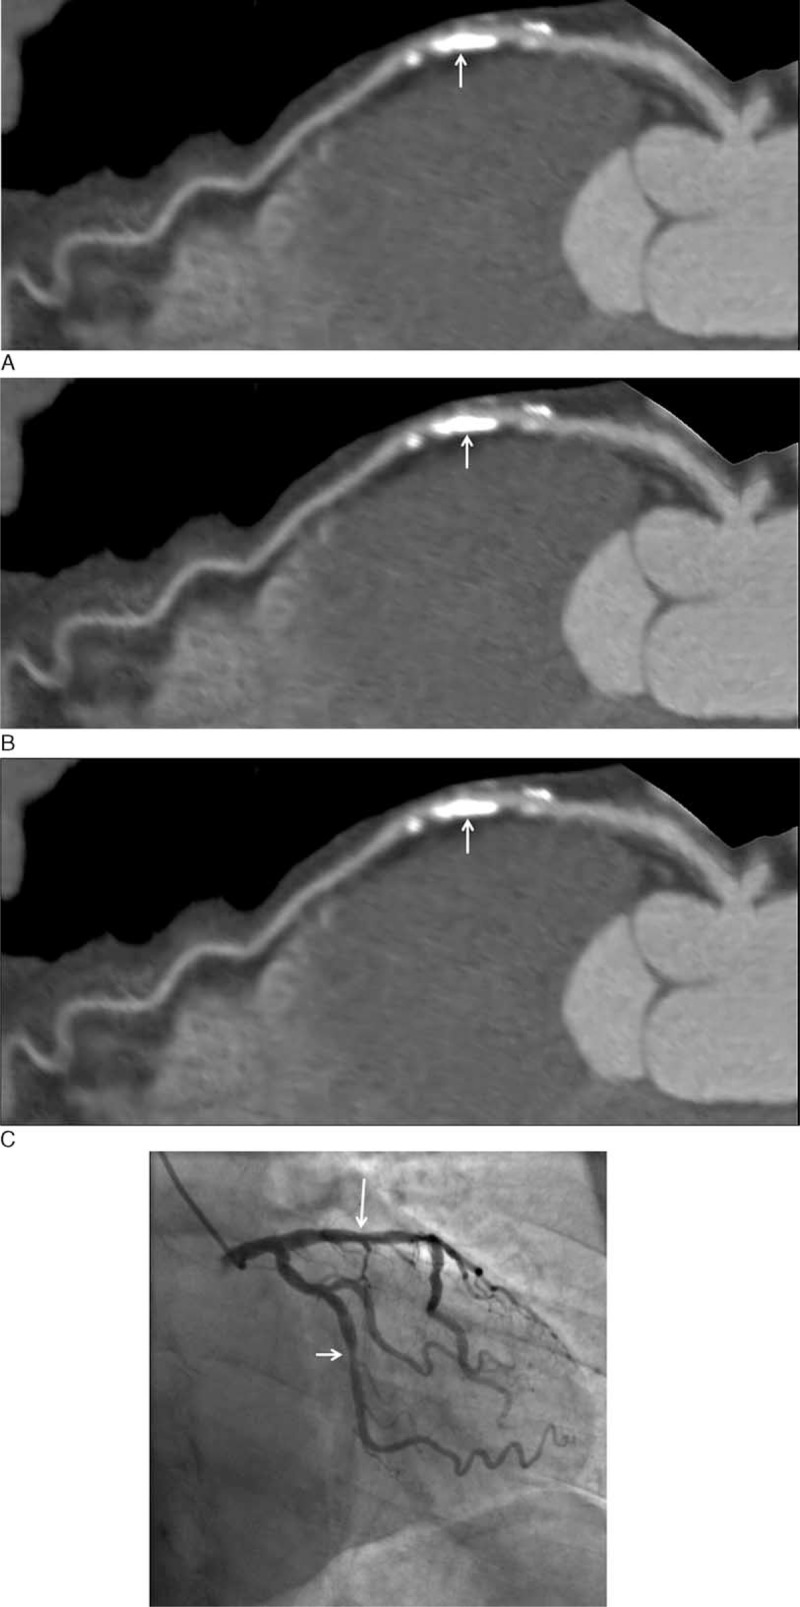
(A) Curved planar reformatted coronary CT angiography (CCTA) shows multiple calcified plaques at the left anterior descending coronary artery (LAD) in a 59-year-old man. CCTA original data (CCTA_O) shows 69% stenosis in LAD due to the heavily calcified plaque, while CCTA with “sharpen” algorithm (CCTA_S) demonstrates 45% stenosis, and 68% stenosis as shown with CCTA with original data integrated with outcome of smoothed image subtracted from the original (CCTA_OS) (A–C). Invasive coronary angiography confirms mild stenosis of 28% at LAD and 44% stenosis at LCx (D). Long and short arrows refer to the stenosis at LAD and LCx, respectively.
